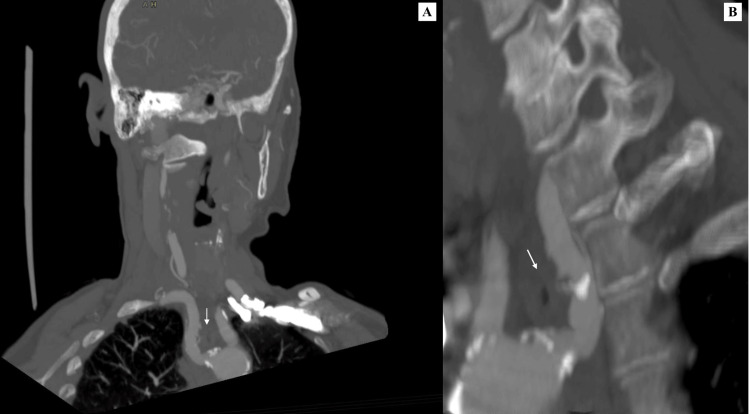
The CTA multi-planar reconstruction (MPR), both coronal (A) and sagittal (B) views, showing the posterior course of the right subclavian artery (white arrow), crossing the mediastinum and compressing the oesophagus. CTA: Computed tomography angiography
